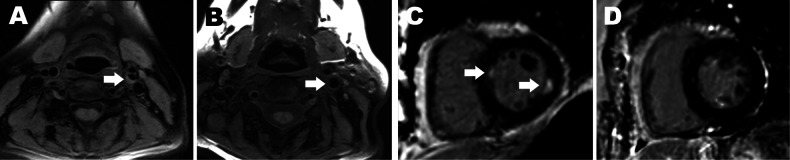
Example of MRI-based assessment of CVD-markers. Atherosclerotic plaque in the left internal carotid artery (arrow, A) compared to a healthy control subject without visualizable atherosclerotic plaque (arrow, B) and subendocardial LGE of the left ventricle (arrows, C) compared to a healthy control subject with no evidence of ischemic enhancement (D)
